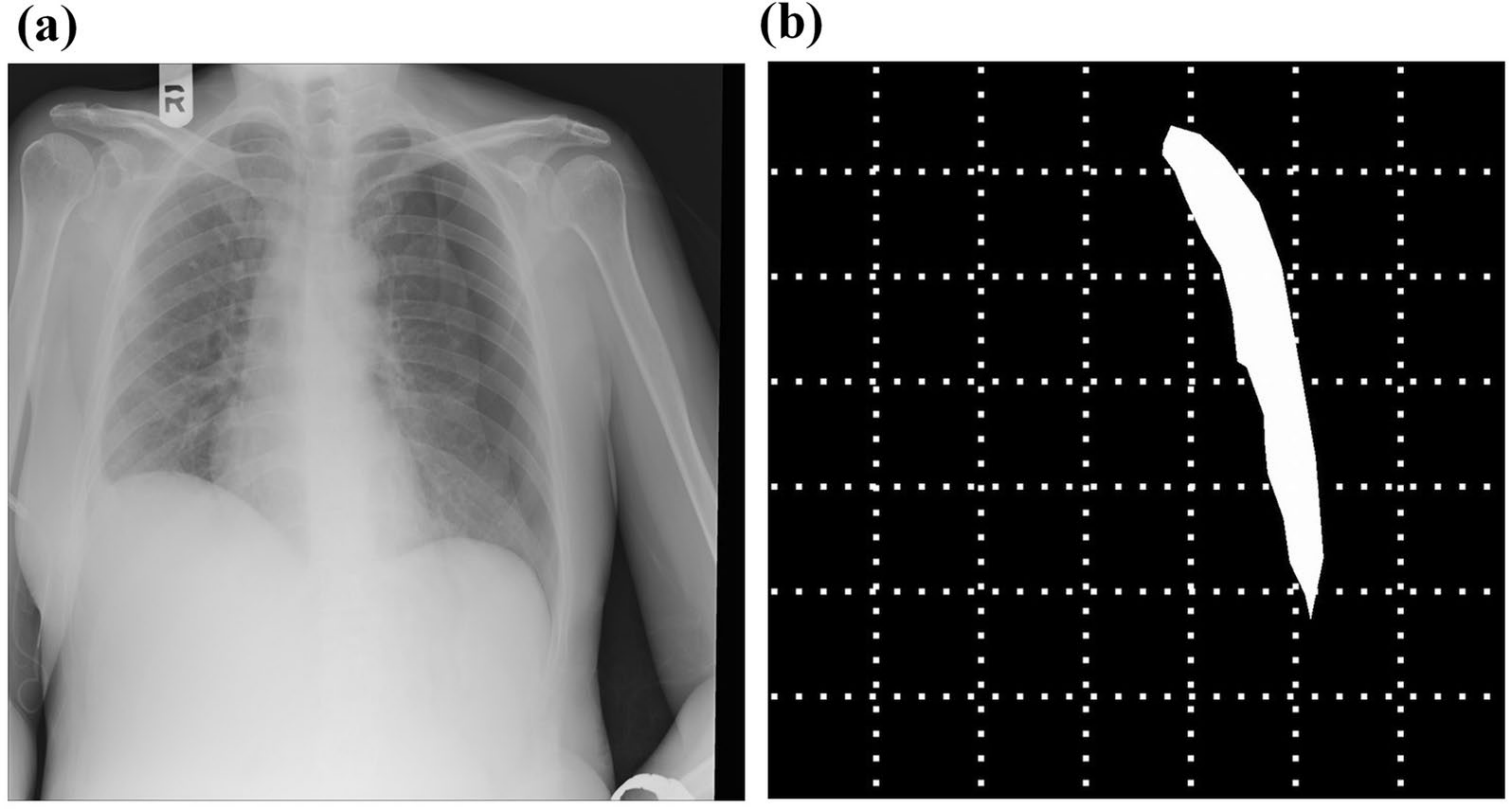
Example of chest X-ray image processing for deep-learning methods. (a) Original chest X-ray image. (b) Image obtained after marking the location of pneumothorax in white and the remaining areas in black.
